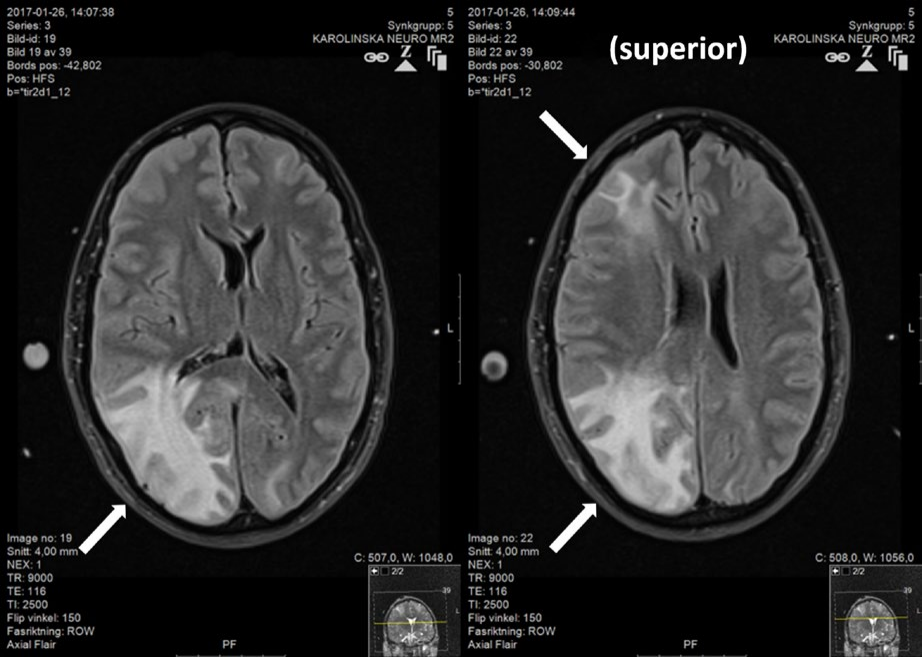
Second magnetic resonance imaging (MRI) after 1 wk (FLAIR images). There were large areas of vasogenic edema in mostly the white matter with expansive effect with compression of sulci over the hemispheres and a left midline shift of 4 mm to the left. There was also vasogenic edema in the superior areas of the brain
